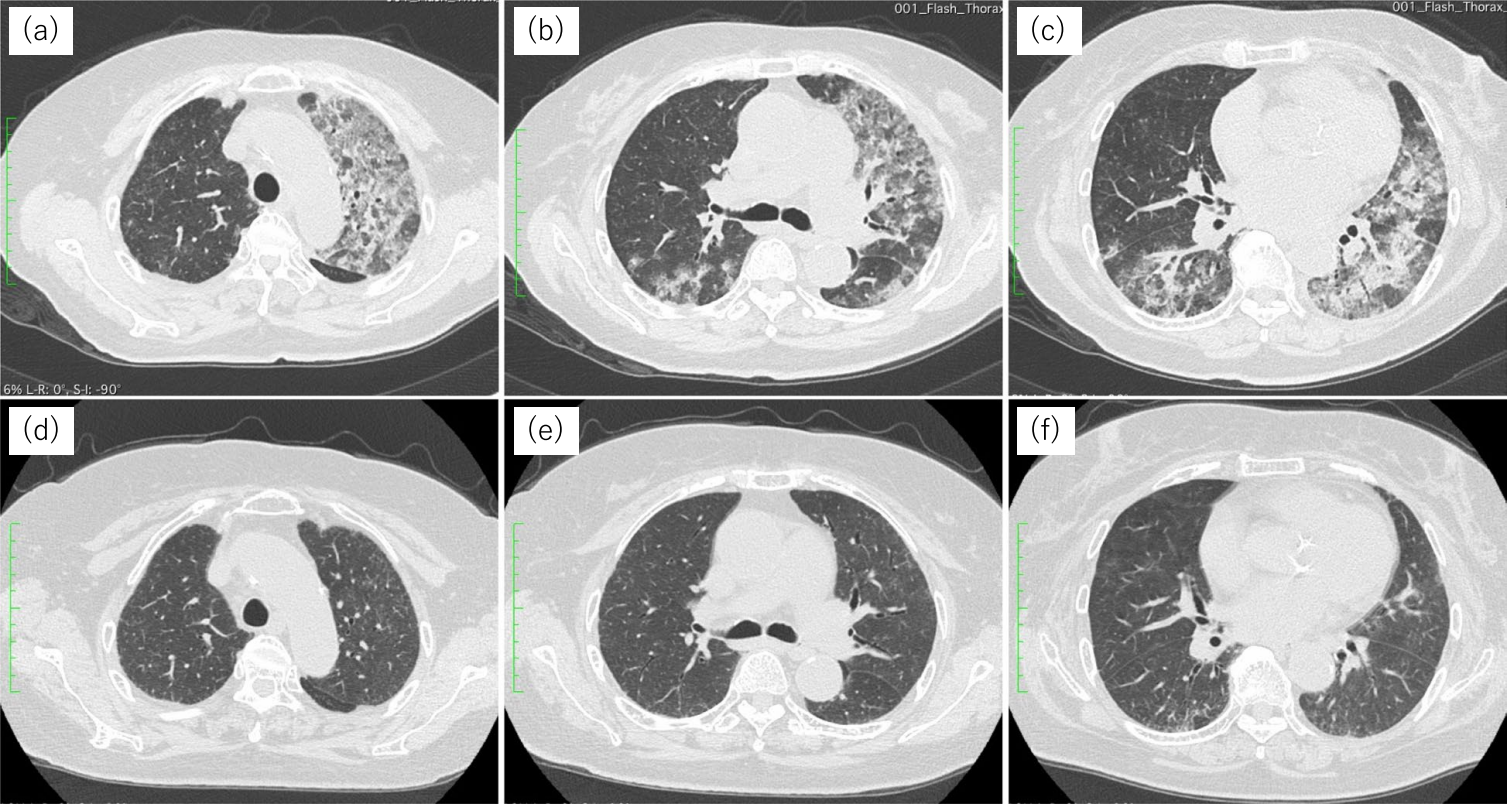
High-resolution computed tomography images of the chest at the time of diagnosis in case 3. The images show frosted shadows and consolidations in the left lung and right lower lobe. The right upper lobe, which exhibits fewer abnormal shadows, shows faint nodular shadows with a central lobular distribution and ground-glass shadows as well. Two months later, a reduction in the shadows is observed (d–f)
